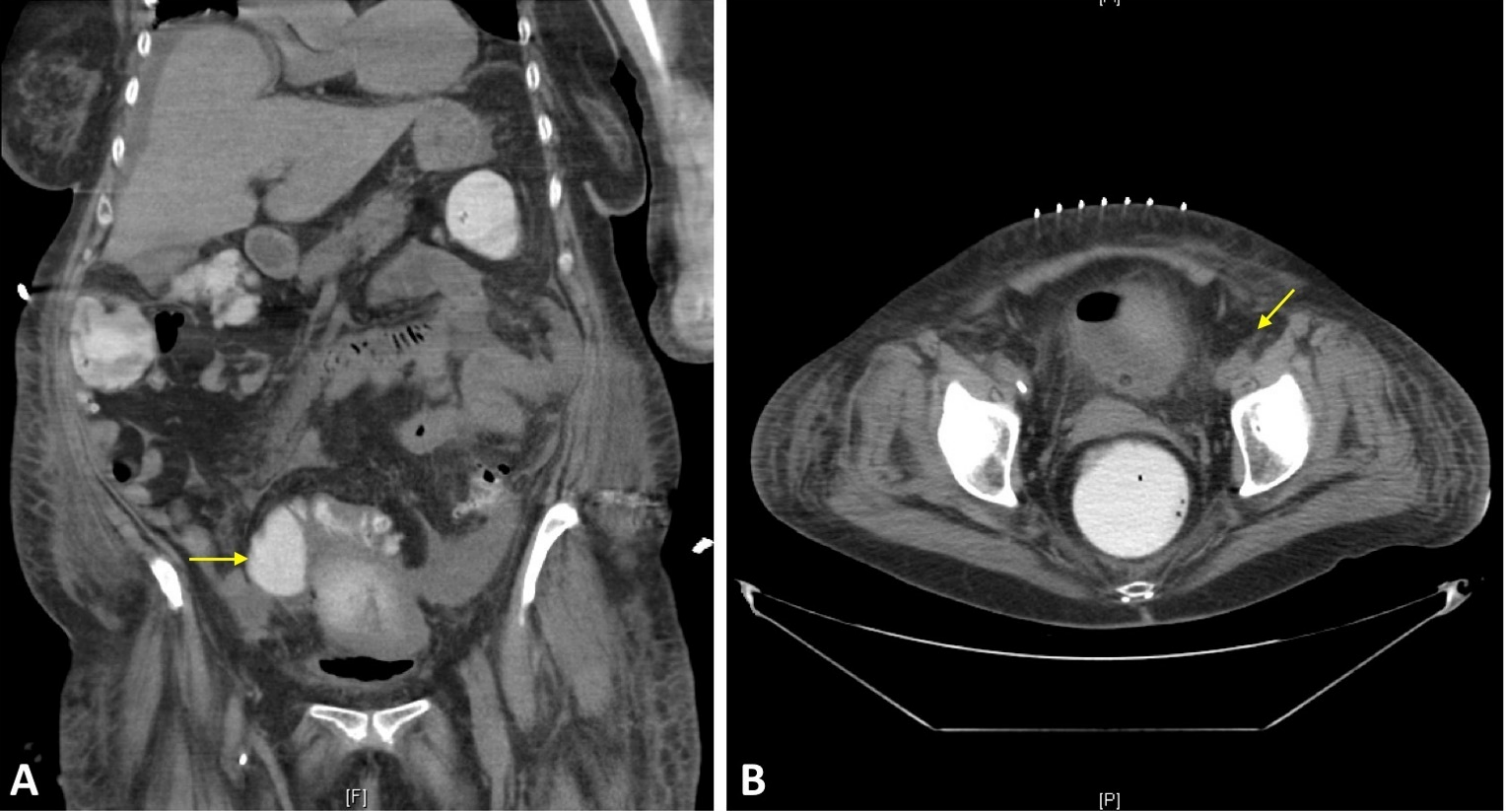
Diverticular Abscess on Computed Tomography (CT) Imaging CT scan showing sigmoid diverticulitis with surrounding inflammation and an adjacent lobulated 6.9 x 5.4 x 6.1 cm abscess (yellow arrow) on (A) coronal and (B) axial sections.
